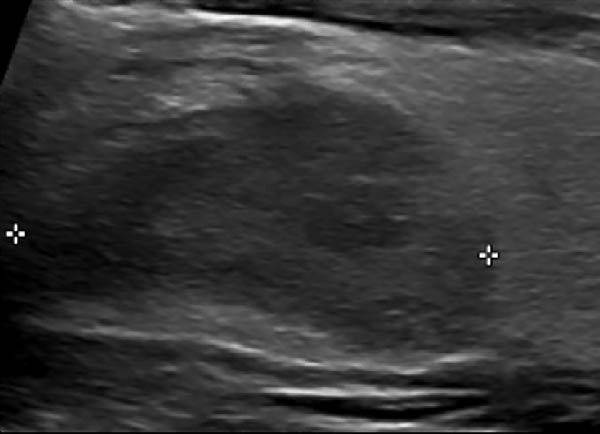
Ultrasound of the neck in longitudinal view showed a 3.3 cm heterogenous mildly hypoechoic avascular nodule abutting the posterior aspect of the left superior thyroid lobe.
